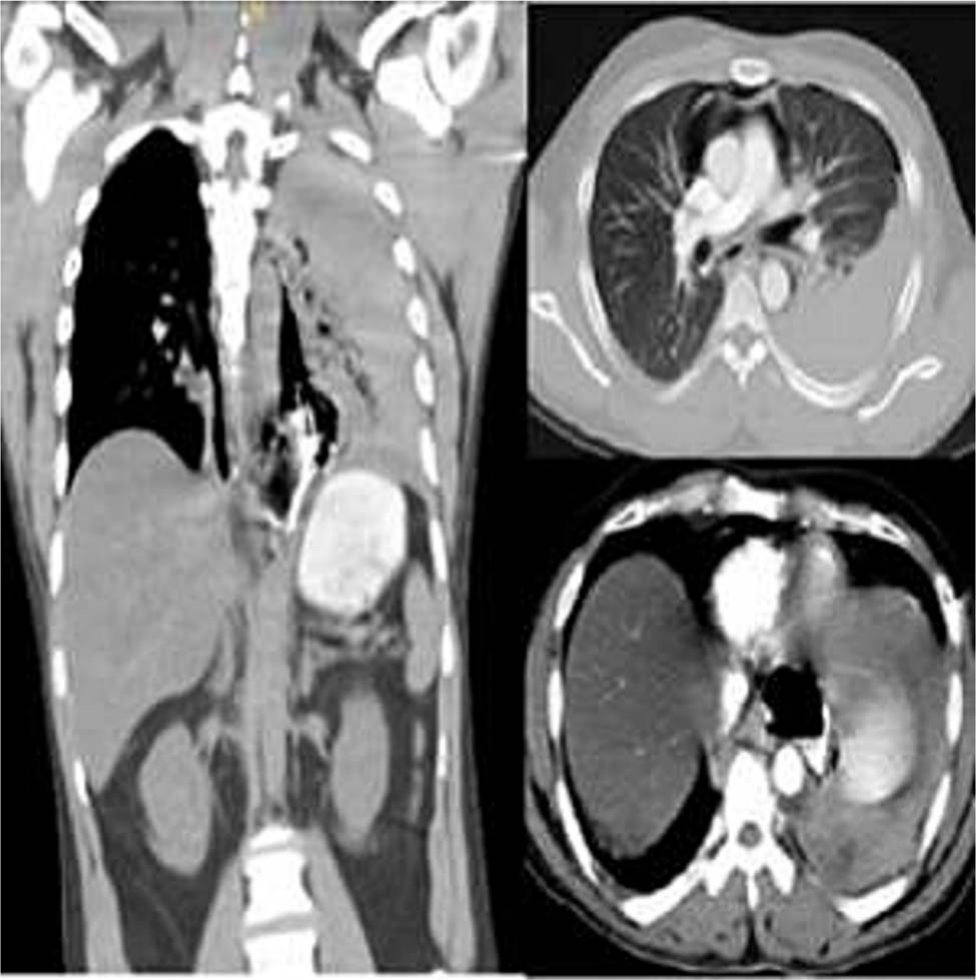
Contrast-enhanced chest CT showing distal esophageal perforation with pneumomediastinum and left hydropneumothorax.
